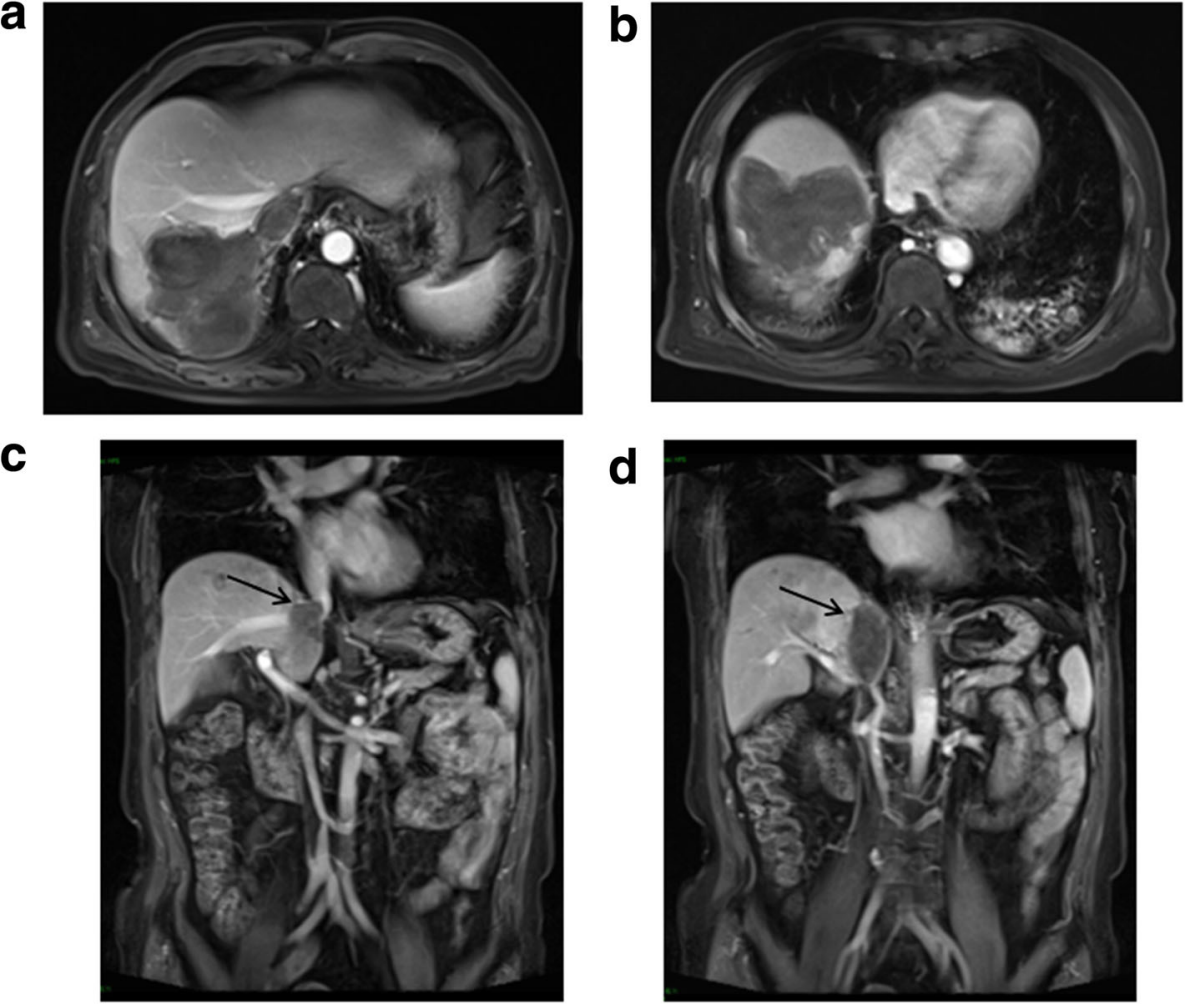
MRI at 1 month post-operatively. a, b Enhanced MRI of the abdominal region showed ablated tumor area at segment VII and VIII. c, d Coronal sequence of enhanced MRI of the abdominal region showed the shrinkage of the tumor thrombus in IVC and RA (*black arrow*). MRI: magnetic resonance imaging, RA: right atrium, IVC: inferior vena cava
